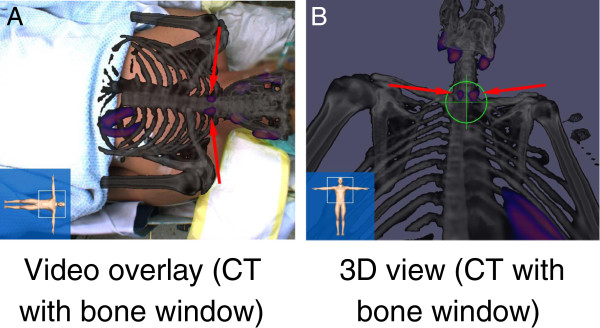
^99m ^Tc-MIBI SPECT/CT of patient 3. Visualized as (A) video overlay on the live video of the patient and in a (B) 3-D virtual reality view of the SPECT/CT from the perspective of the gamma probe. The arrows show the parathyroid adenomas, and in (B), the target shows the direction the gamma probe is pointing at the time the screenshot was captured.
